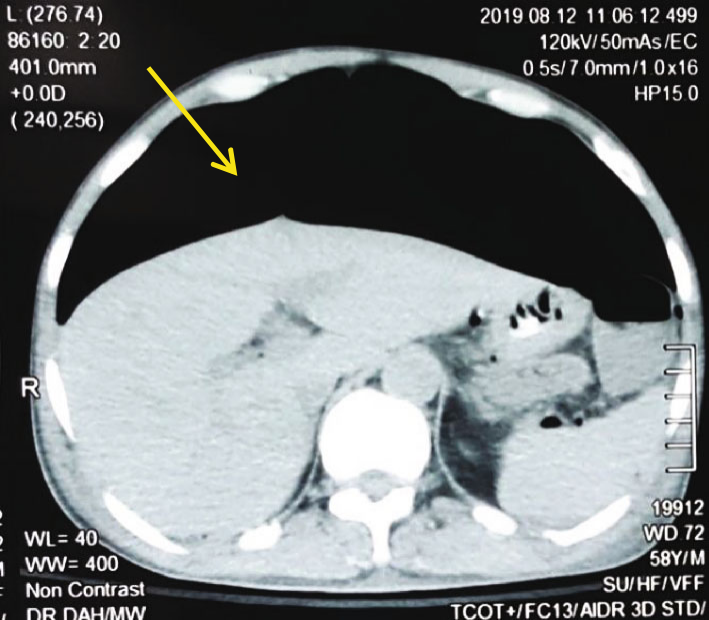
Noncontrast CT scan of the abdomen: yellow arrow shows the presence of intraperitoneal gas.
